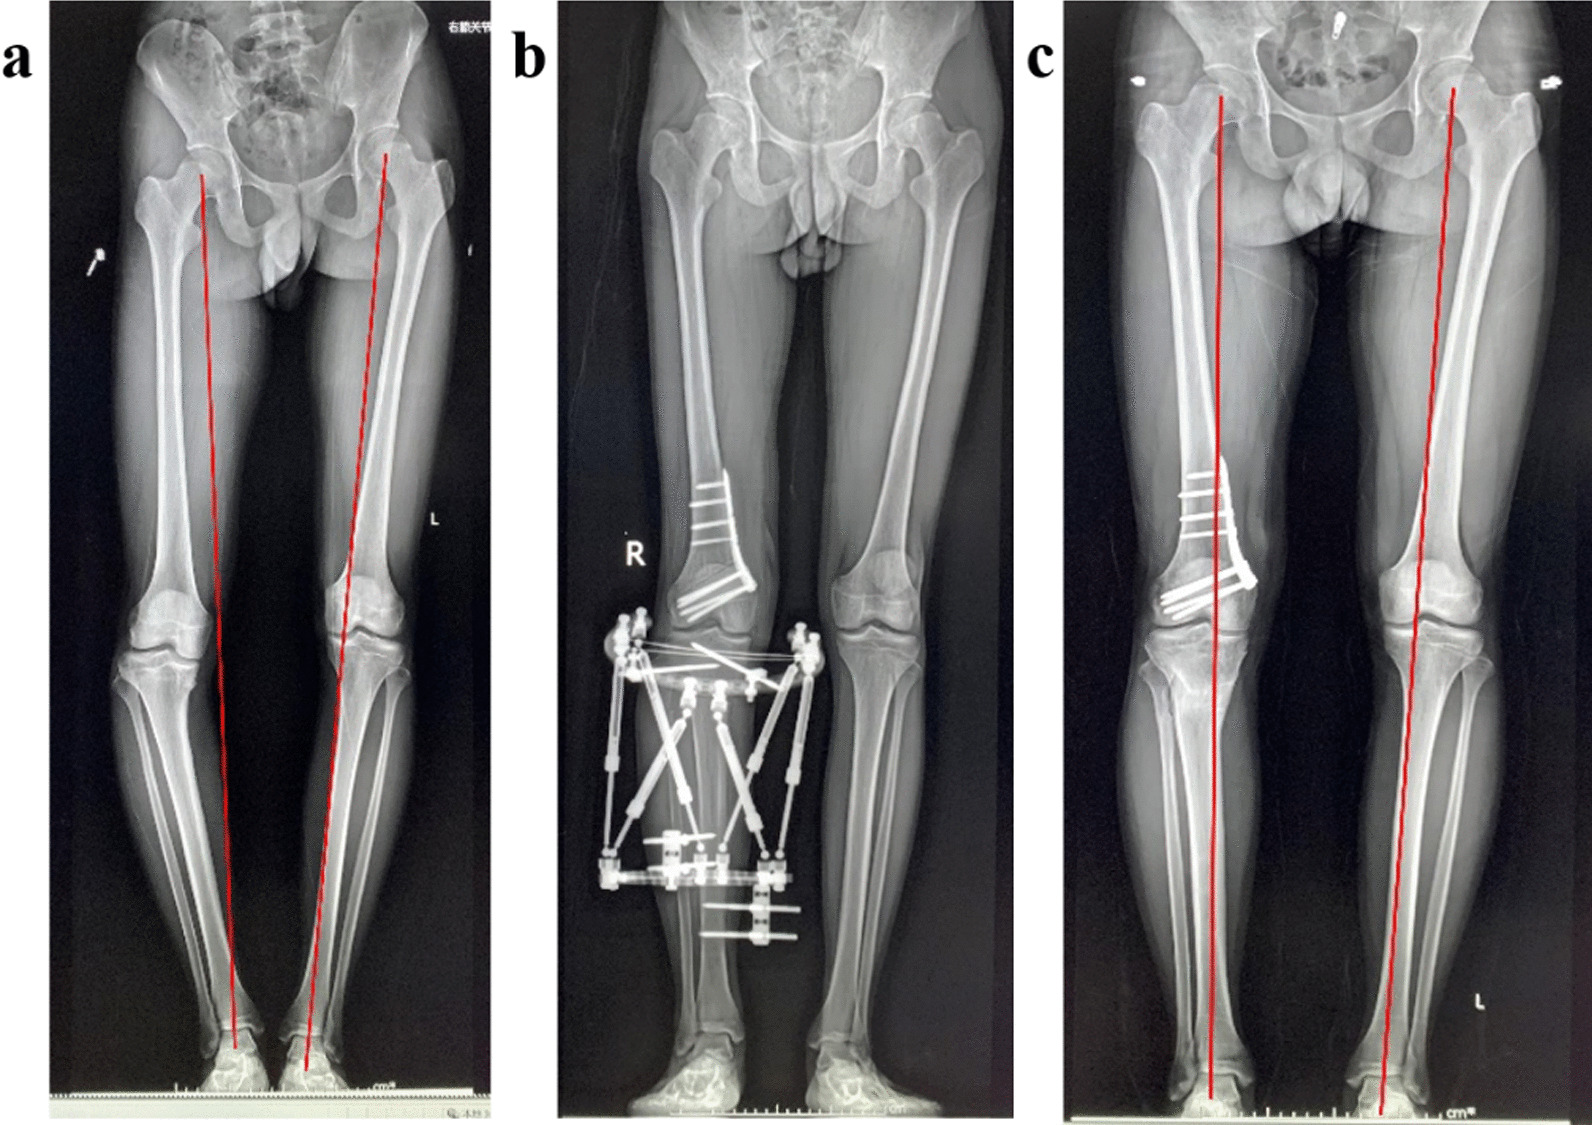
An 18-year-old patient had right varus knee because of Blount disease. The right lower limb was 2 cm shorter than the left. a Full-length radiograph before operation. b X-ray after operation shows DFO was, and QSF was applied. Re-examination X-ray shows the osteotomy site healed well, and the QSF was removed. The lower limb alignment of right limb and the LLD is near normal
